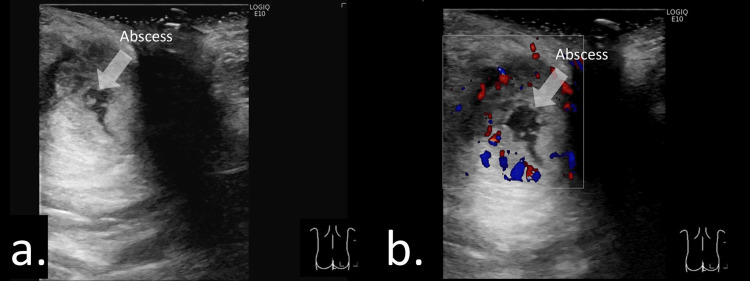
Inflammatory bowel disease presenting with an anal ulcer and pain at the 12 o’clock position in a 12-year-old female. (a, b) Sagittal sonograms demonstrating a small hypoechoic abscess measuring 9 mm, surrounded by reactive hypervascular changes.
